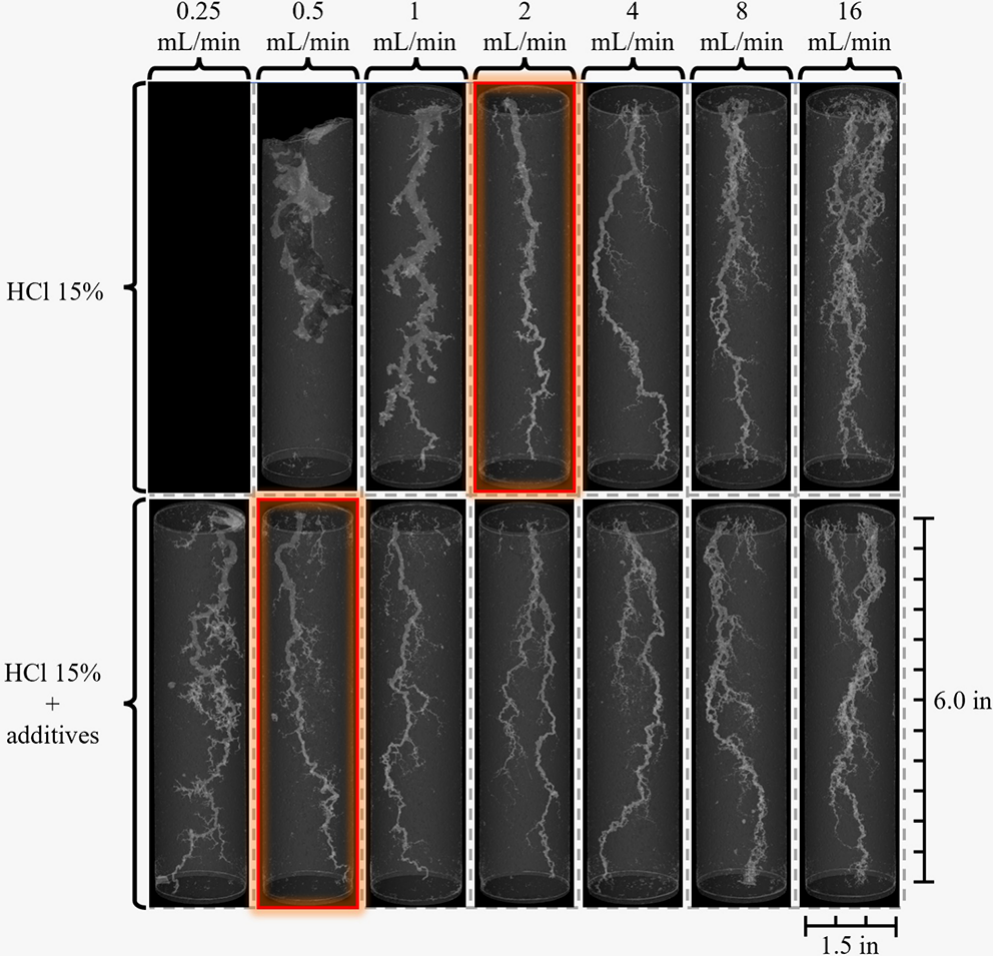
X-ray microcomputed tomography
